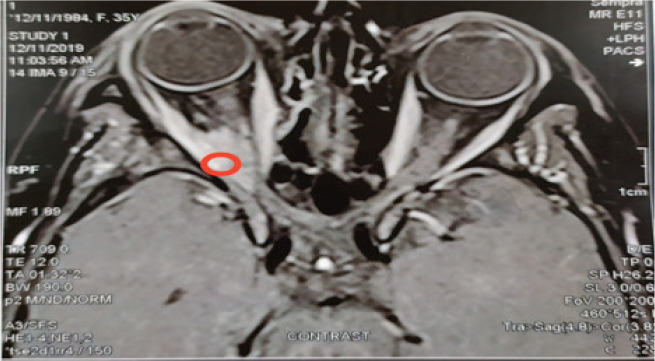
MRI brain at dignosis: T1 with contrast shows right superior rectal muscle thickening about 14 mm associated with slight proptosis of the right eye.
